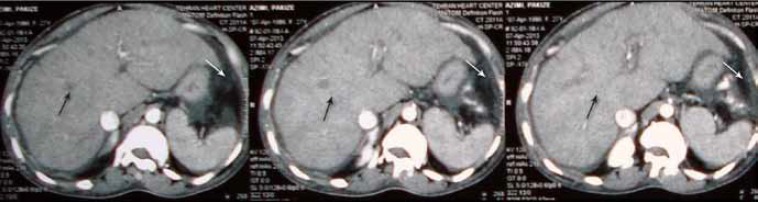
Abdominal CT-scan shows mild hepatomegaly (black arrows) and ascites (white arrows).
